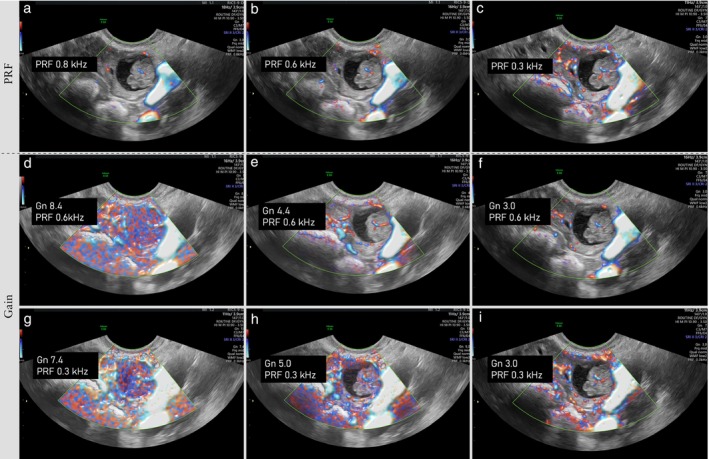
Doppler settings. Power Doppler ultrasound images of a unilocular‐solid cyst with a papillary projection obtained with different pulse repetition frequency (PRF) and power Doppler gain (Gn): (a–c) fixed gain (Gn, 3.0) and decreasing PRF, from 0.8 kHz (a) to PRF 0.6 kHz (b) to PRF 0.3 kHz (c); (d–f) fixed PRF (PRF, 0.6 KHz) and decreasing Gn, from 8.4 (d) to 4.4 (e) to 3.0 (f); (g–i) fixed PRF (PRF, 0.3KHz) and decreasing Gn, from 7.4 (g) to 5.0 (h) to 3.0 (i). See also Videoclip S20 for more details.
